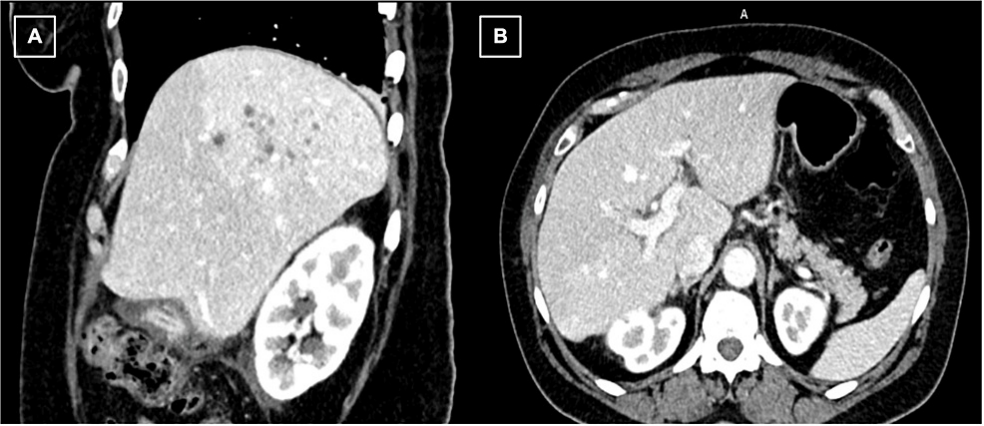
Sagittal (A) and axial (B) CT abdomen and pelvis showing edematous gallbladder, hepatic abscesses, and dilated intrahepatic bile ducts
